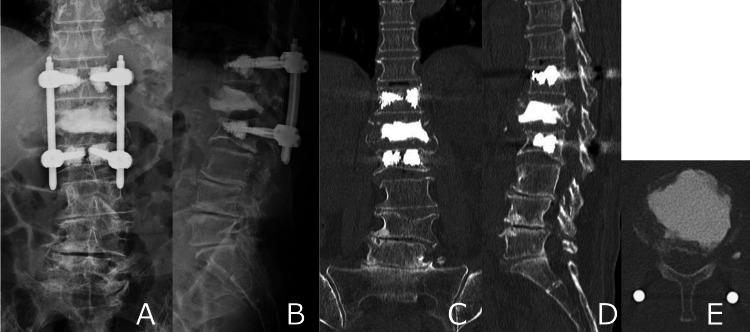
Postoperative imaging. (A–B) X-ray and (C–E) computed tomography (CT) images showing T12–L2 fixation with calcium phosphate cement and cement augmentation of pedicle screws.
